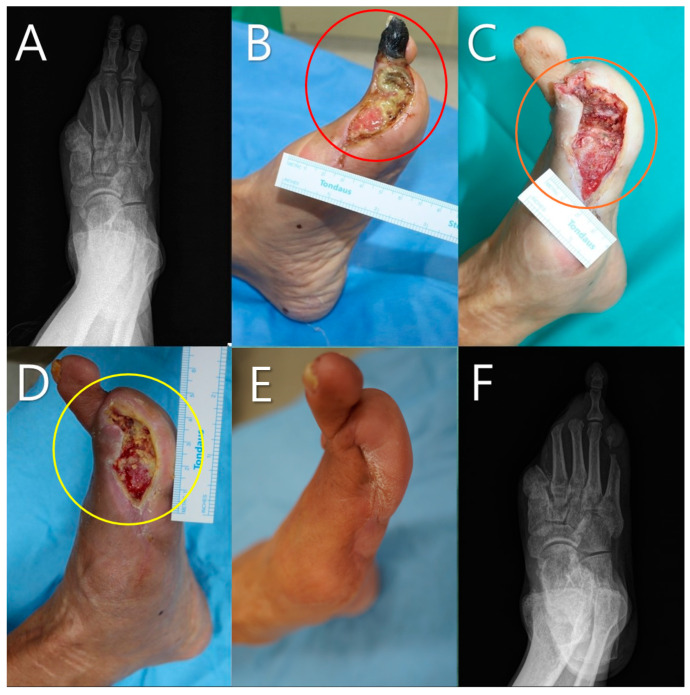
A 62-year-old male with a history of hypertension and diabetes. (A) Initial X-ray showing the extent of necrosis. (B) Pre-debridement image showing necrotic changes (red circle). (C) Post-debridement image showing the wound bed and poor tissue quality around the wound due to poor perfusion (orange circle). (D) Wound after 9 days following a total of four mADM applications, showing granulation tissue growth (yellow circle). (E) Wound after 12 weeks, showing successful secondary healing. (F) Final X-ray showing no signs of gas gangrene, pus accumulation, or osteomyelitis.
